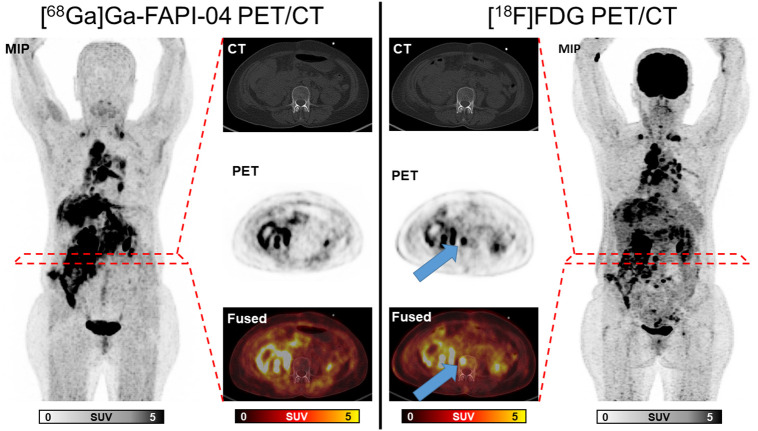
Maximum-intensity projection (MIP), CT, PET, and fused images of [^68^Ga]Ga-FAPI-04 PET/CT and [^18^F]FDG PET/CT of patient 2. Images show concordant high uptake in multiple lymph node metastases and advanced peritoneal carcinomatosis. In addition, [^18^F]FDG PET shows osseous metastases not seen on [^68^Ga]Ga-FAPI-04 PET (arrows). Time difference between scans was 1 d.
